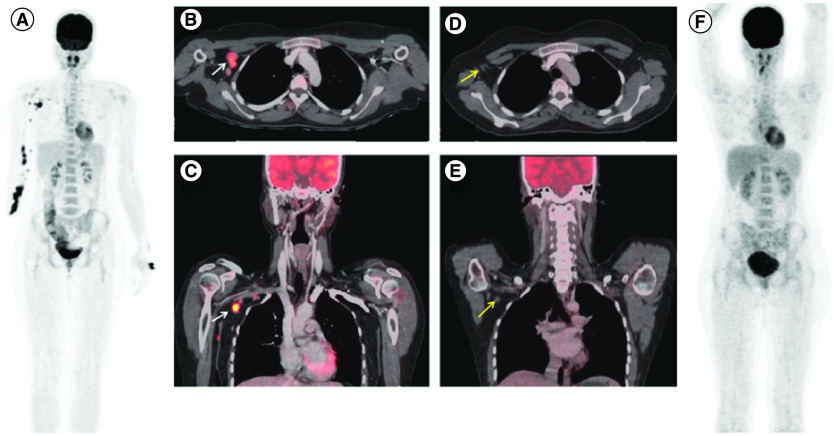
A 29-year-old female presented with history of recurrent soft tissue mass in right hand and had multiple surgeries. ^18^F-FDG positron emission tomography/computed tomography (PET/CT) scan was performed in August 2020. (A) MIP image showed multiple FDG avid soft tissue density deposits in subcutaneous and muscular planes of right upper limb along with right axillary lymph nodes. (B) Axial and (C) coronal cross section images showed enlarged FDG avid right axillary lymph nodes (white arrows). After chemotherapy, 18F-FDG PET/CT was done to assess response in May 2021. (D) Axial and (E) coronal images shows reduction in size of axillary lymph nodes (yellow arrow) with no significant FDG uptake. (F) Maximum intensity projection image showed metabolic resolution of lesions previously observed, suggesting complete metabolic response.
